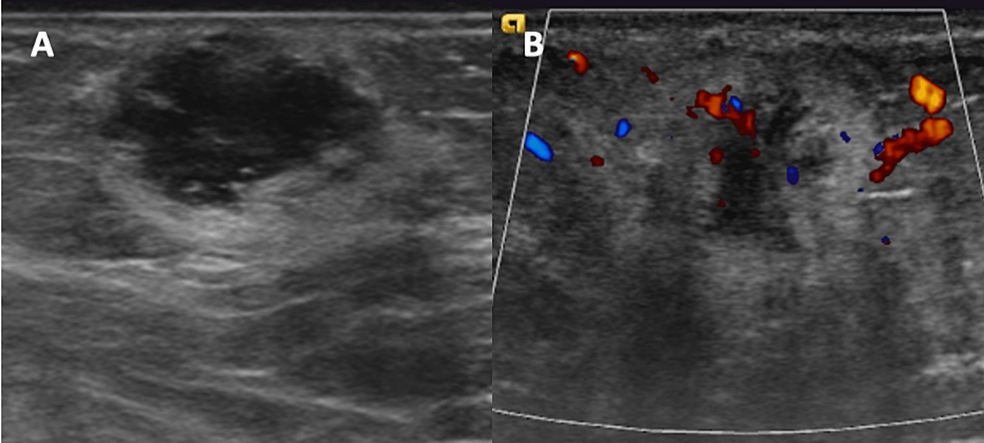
A) Ultrasound of the left breast showing a hypoechoic, irregular mass with irregular margins and B) color Doppler ultrasound showing associated echogenic rim and internal vascularity at the mammographic area of concern
